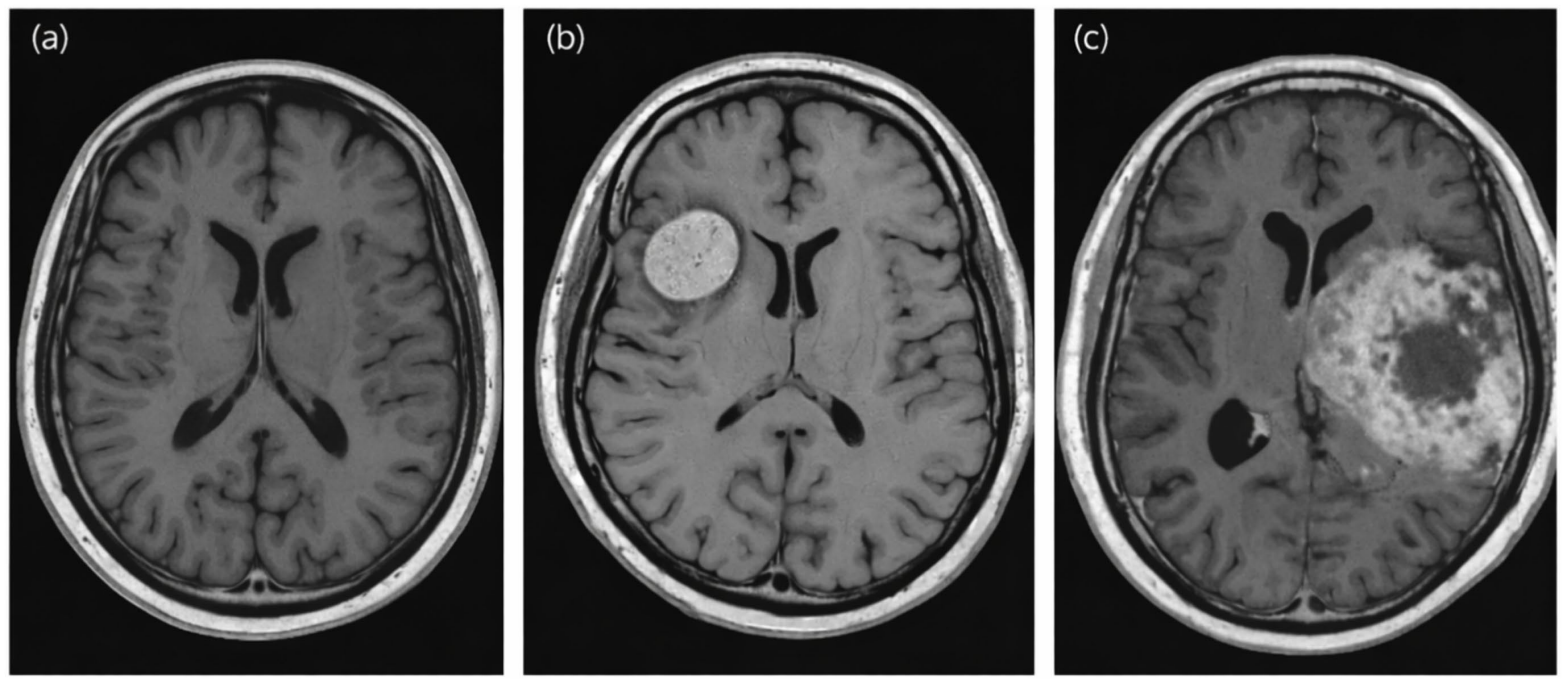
Representative MRI images of normal brain tissue (a), benign tumors (b), and malignant tumors (c). Normal anatomy is symmetric without focal lesions. Benign tumors appear well circumscribed with minimal edema. Malignant tumors typically exhibit irregular, infiltrative margins, heterogeneous signal intensity, and mass effect (Karagoz et al., 2024). Licensed under the terms and conditions of the Creative Commons Attribution (CC BY) license (https://creativecommons.org/licenses/by/4.0/).
